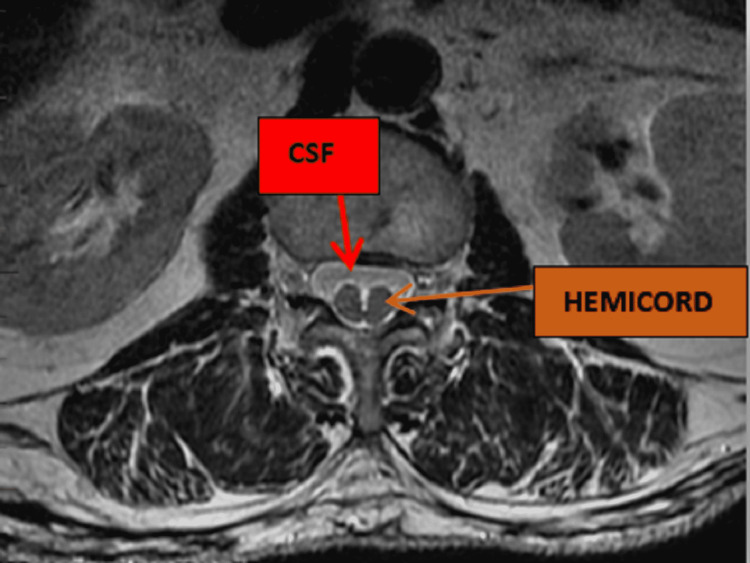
T2-weighted axial lumbar MRI showing two separate hemicords at the level L2-L3.
